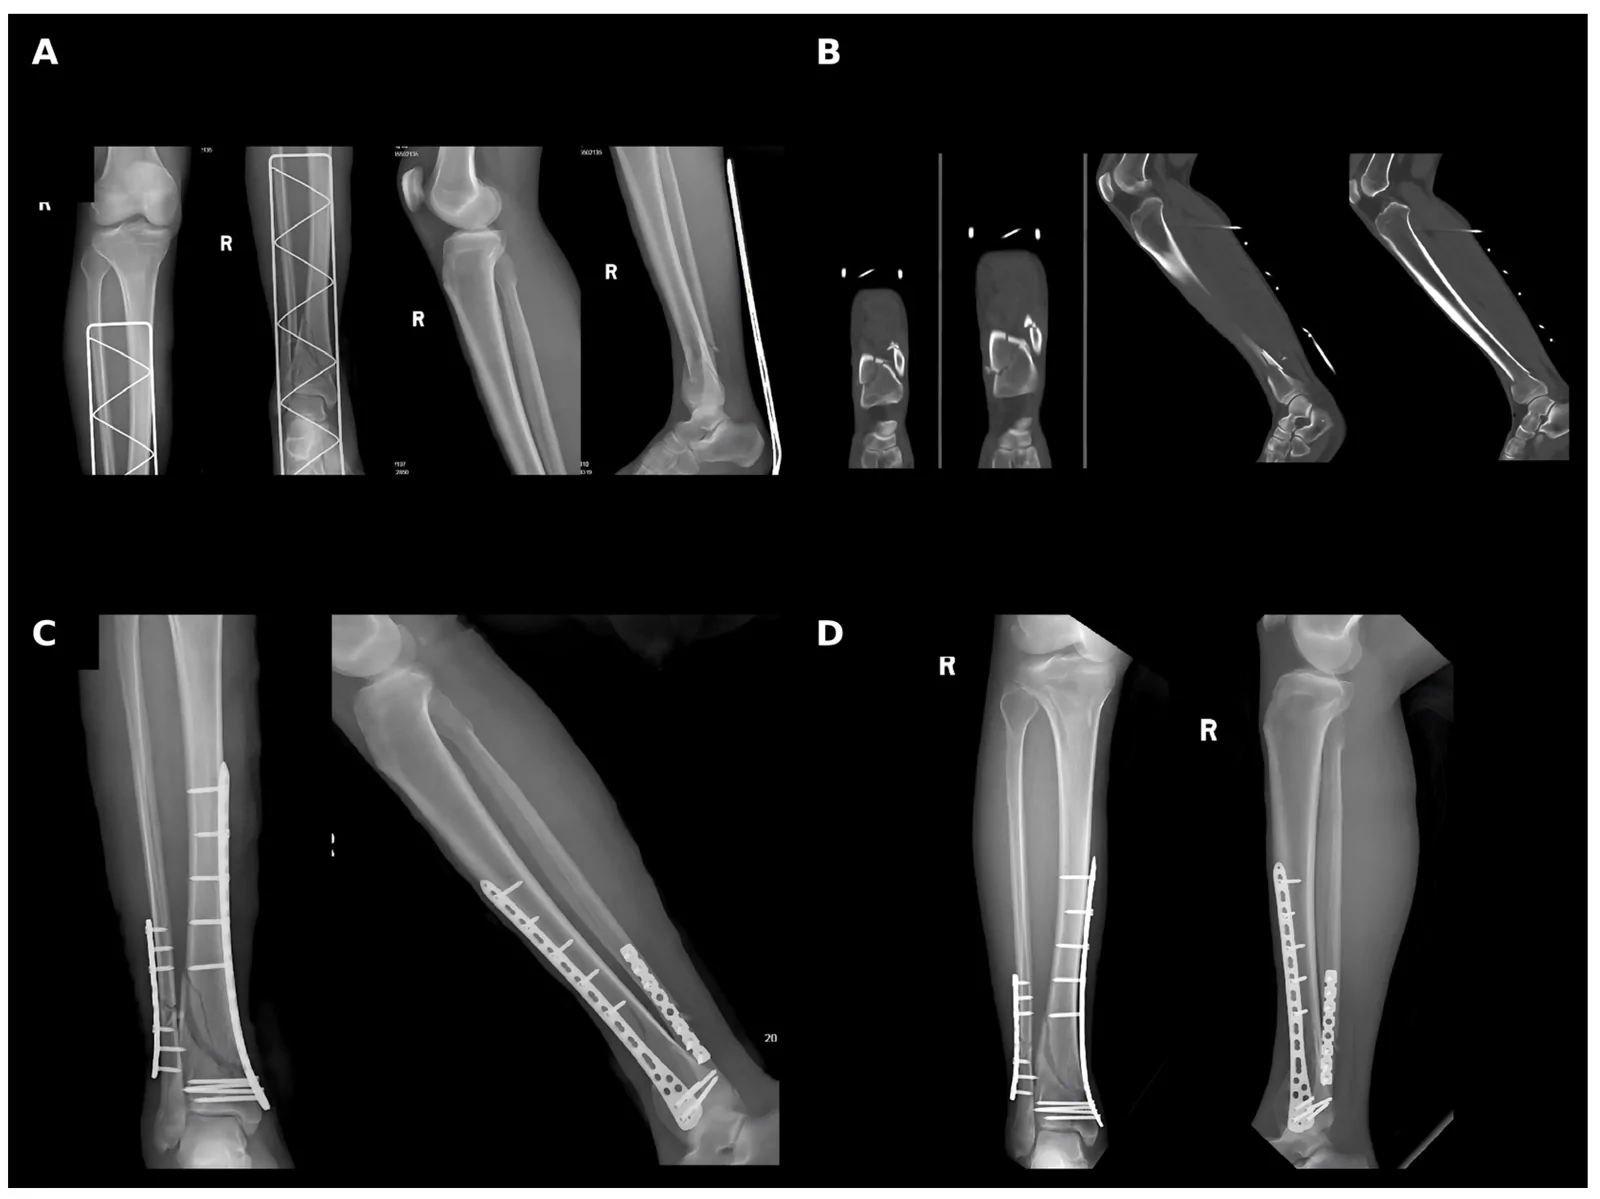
Representative case treated with plate fixation. (A) Preoperative anteroposterior and lateral radiographs showing a distal tibial fracture; (B) preoperative computed-tomography images demonstrating fracture morphology; (C) postoperative anteroposterior and lateral radiographs after distal tibial plate fixation with supplemental fibular fixation; (D) follow-up radiographs showing maintained implant position and alignment with interval fracture healing. R, right side.
